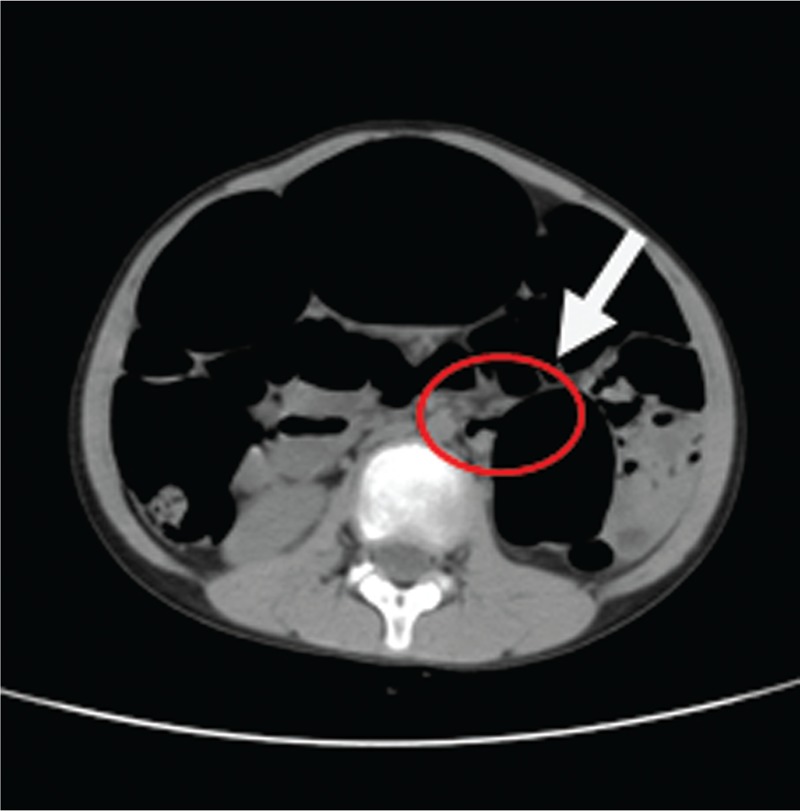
Transition point with an abrupt reduction in bowel caliber seen as a “beak” or “ace of spades” sign in abdominal CT (arrow).
